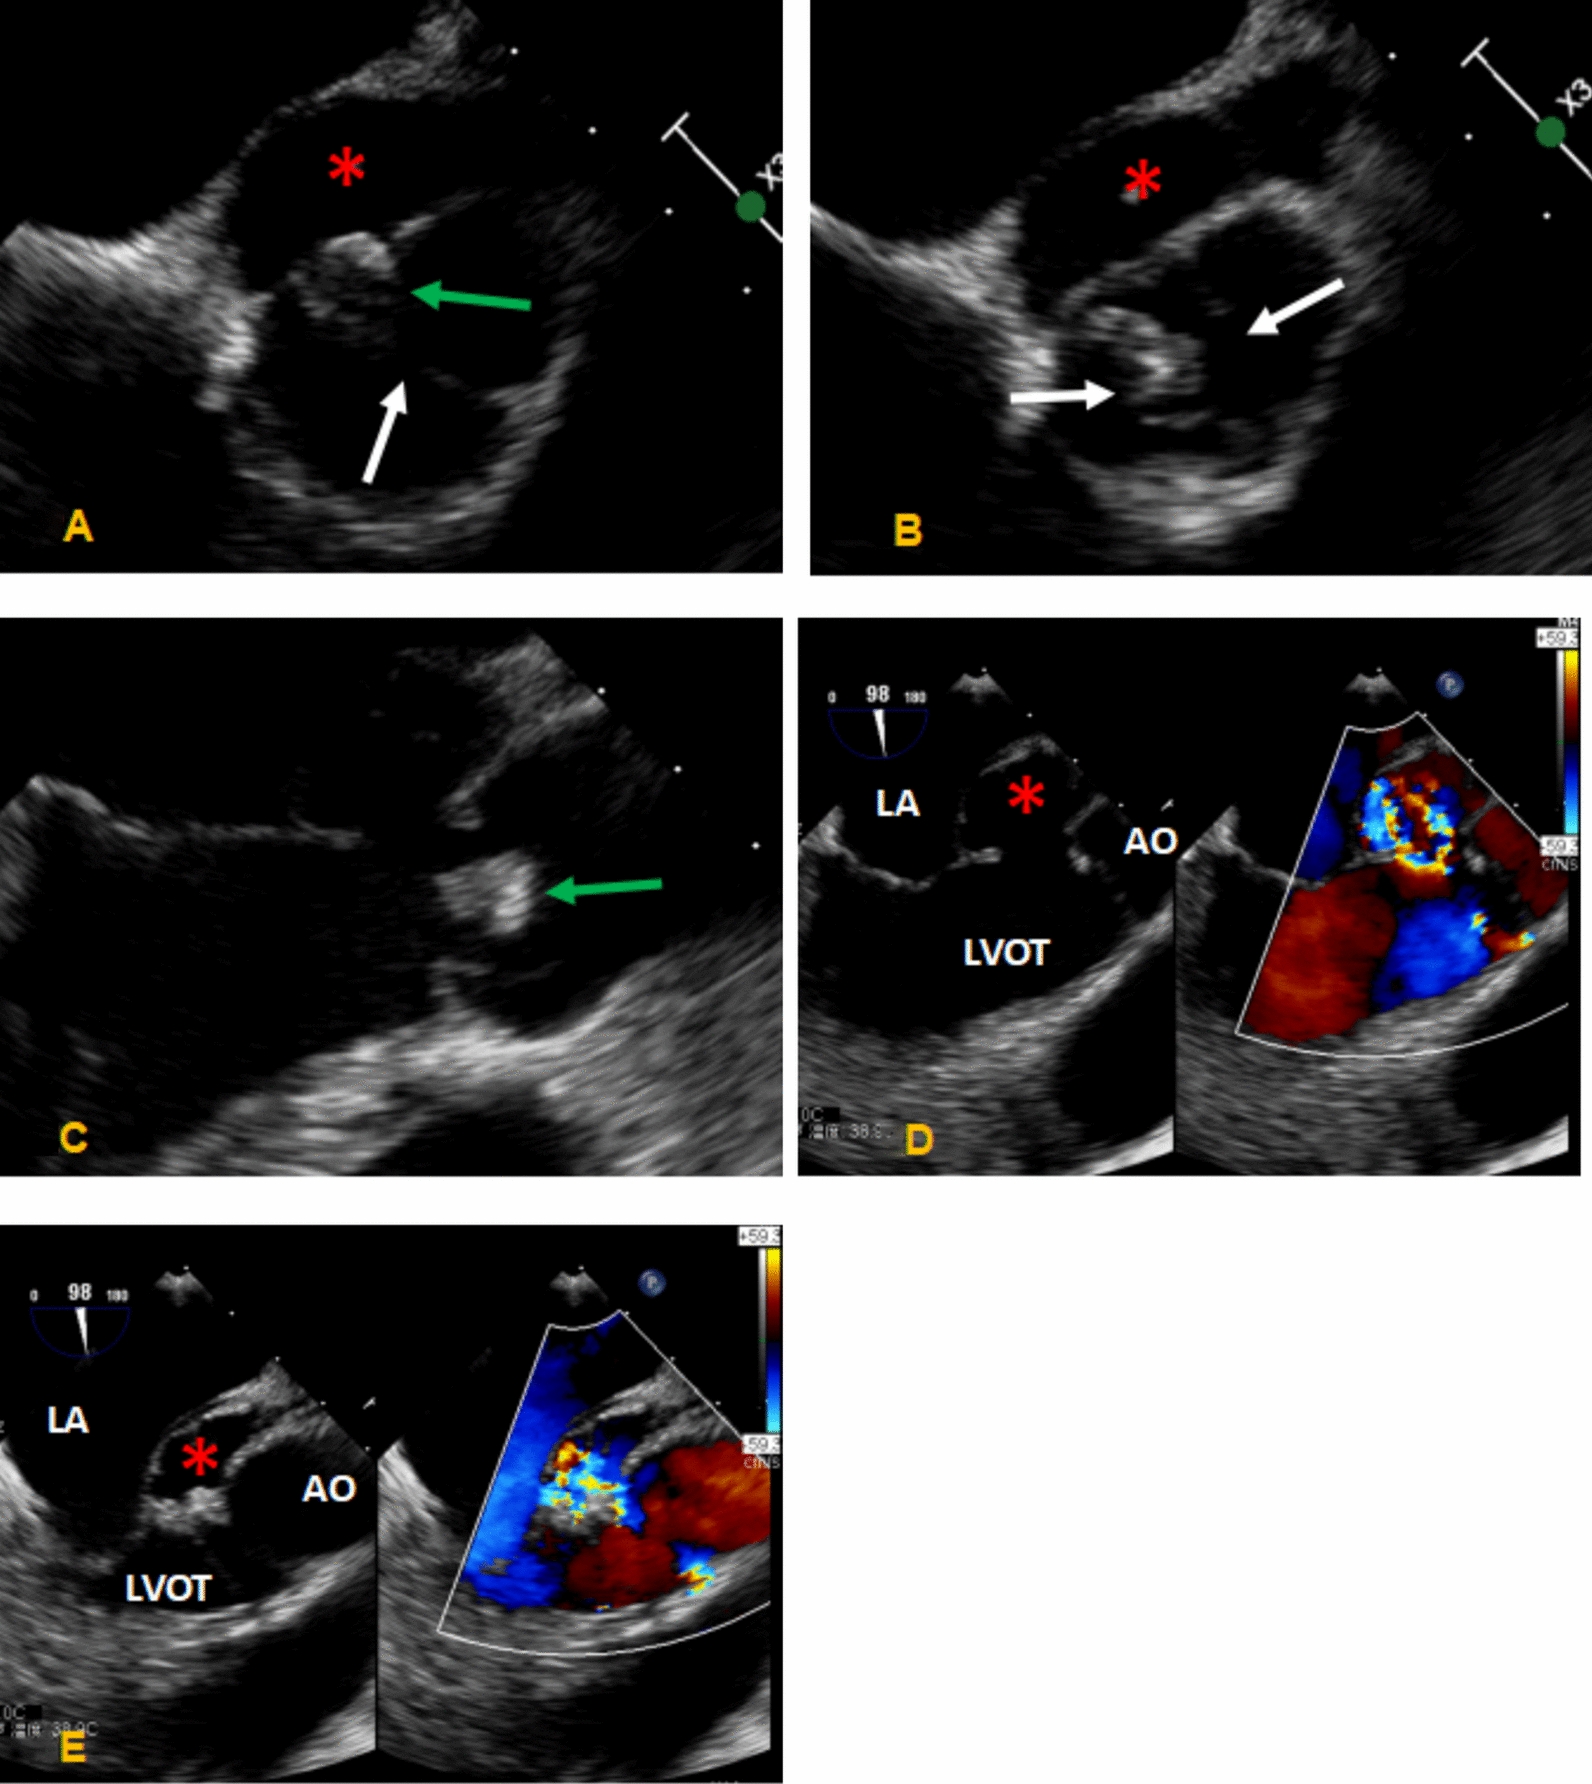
Transesophageal echocardiography. A, B Detailed view of the bicuspid valve showing "I" and "II" patterns during the cardiac cycle. C Vegetations on the leaflet (green arrow). D Systolic expansion of the pseudoaneurysm (asterisk) communicating with the LVOT. E Diastolic collapse of the cavity (asterisk)
